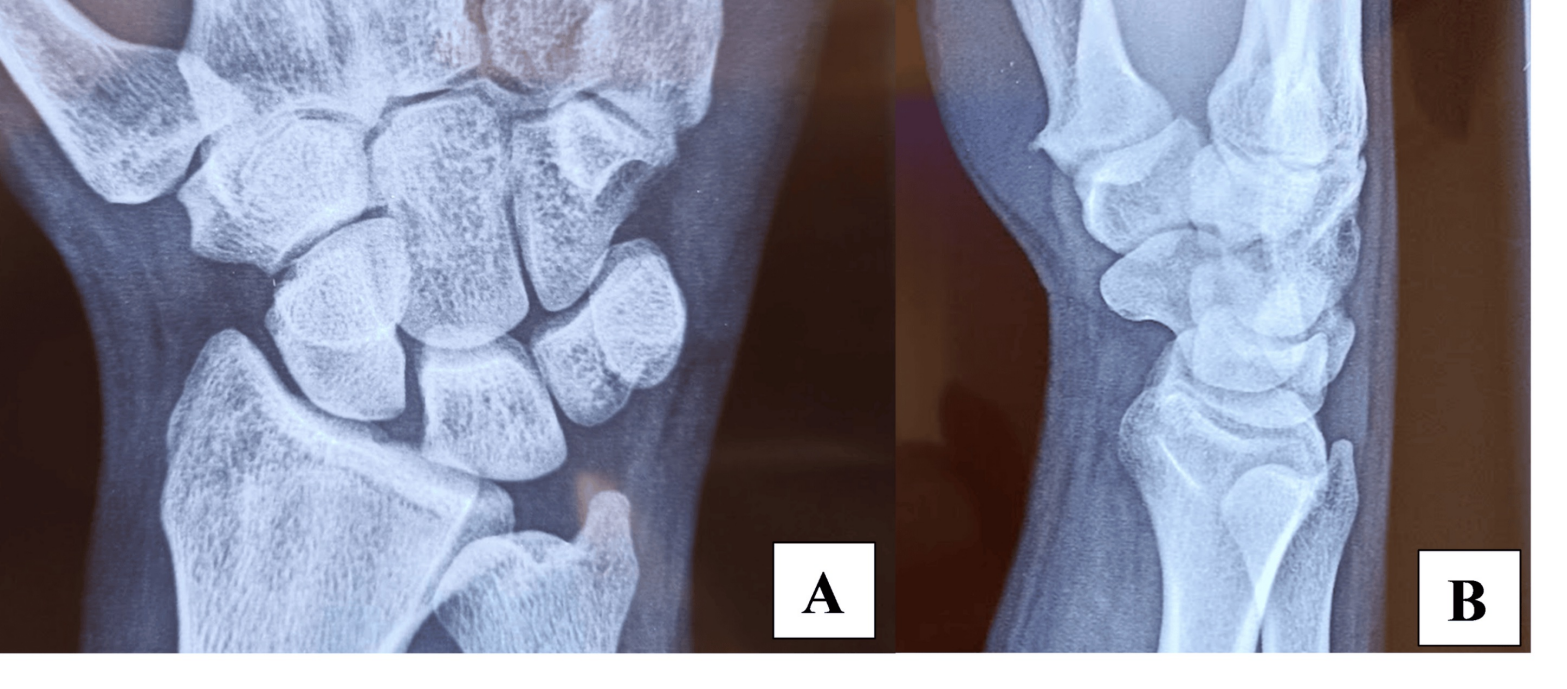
X-ray imaging of the wrist in anteroposterior (A) and lateral (B) views, showing normal bone alignment with no fractures or dislocation.
